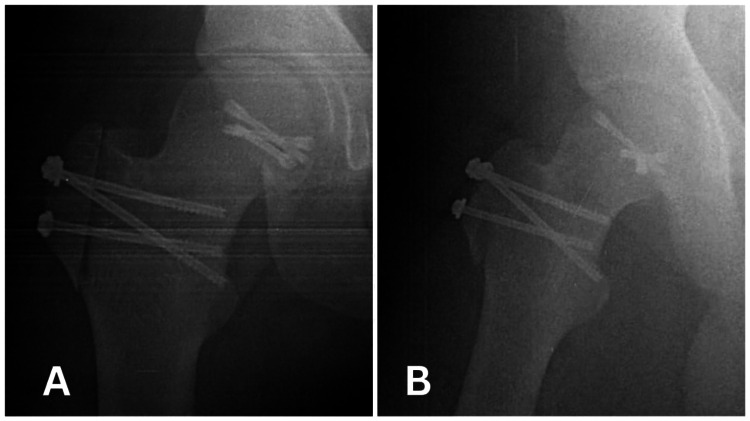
Initial post-operative day 0 radiograph of operated right hip. (A) Anteroposterior view. (B) Oblique view. Both views demonstrate (i) anatomical reduction of the femoral head fragment held by three 4 mm Herbert headless compression screws — two of 4 mm × 18 mm placed antegrade through the fragment, and one of 4 mm × 24 mm placed retrograde from the parent femoral head as a supplementary lag screw — all three crossing the fracture plane and countersunk beneath the articular cartilage; and (ii) anatomical reduction of the greater trochanteric flip osteotomy held by three definitive 3.5 mm cortical screws after replacement of the provisional Kirschner wires.
